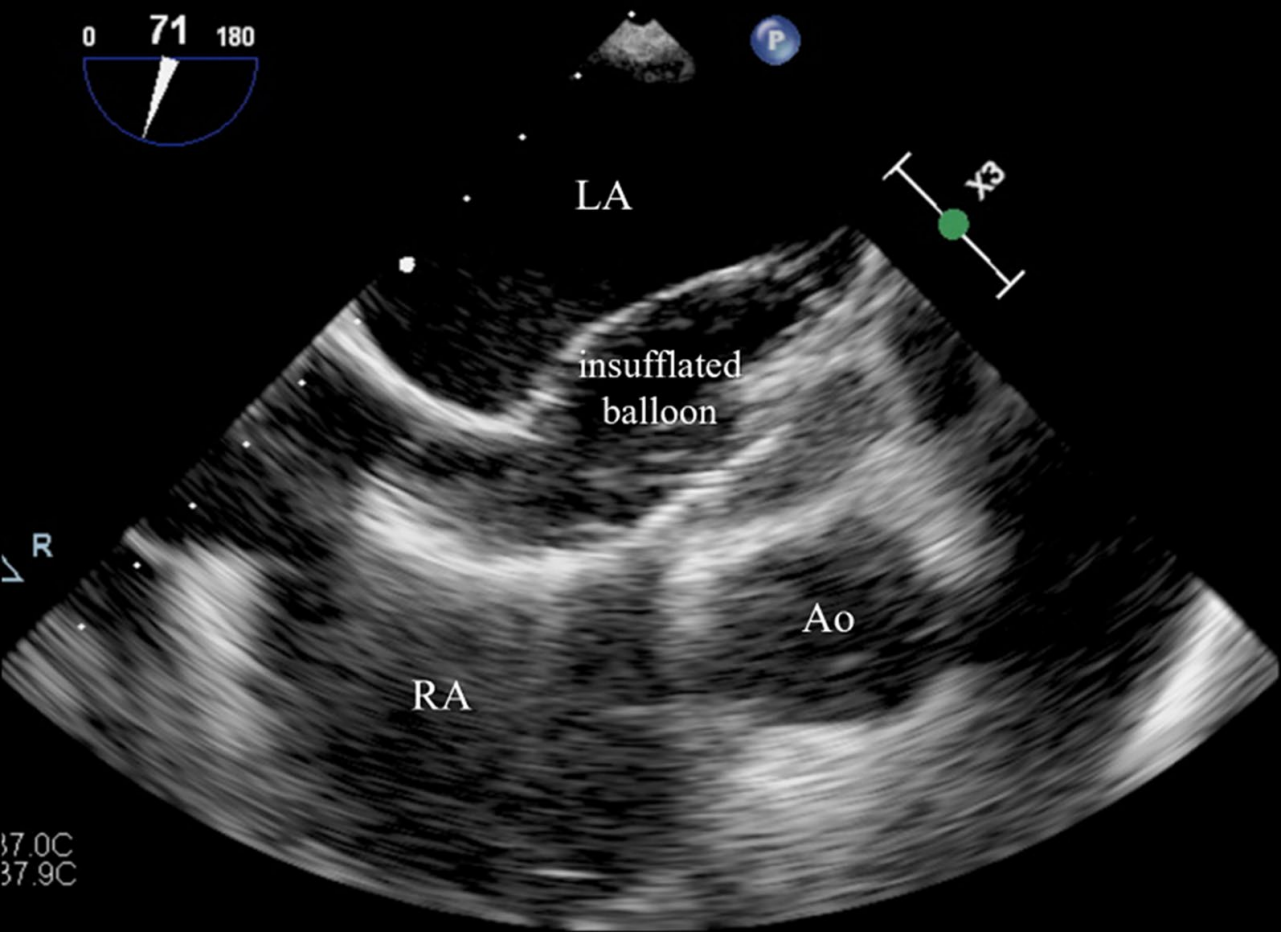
Temporary balloon occlusion test of the ASD with TEE guidance. Before permanent percutaneous ASD closure, a temporary balloon occlusion test was performed in order to check for the increase in pulmonary pressures. *Ao* aorta, *ASD* atrial septal defect, *LA* left atrium, *RA* right atrium, *TEE* trans-esophageal echocardiography
